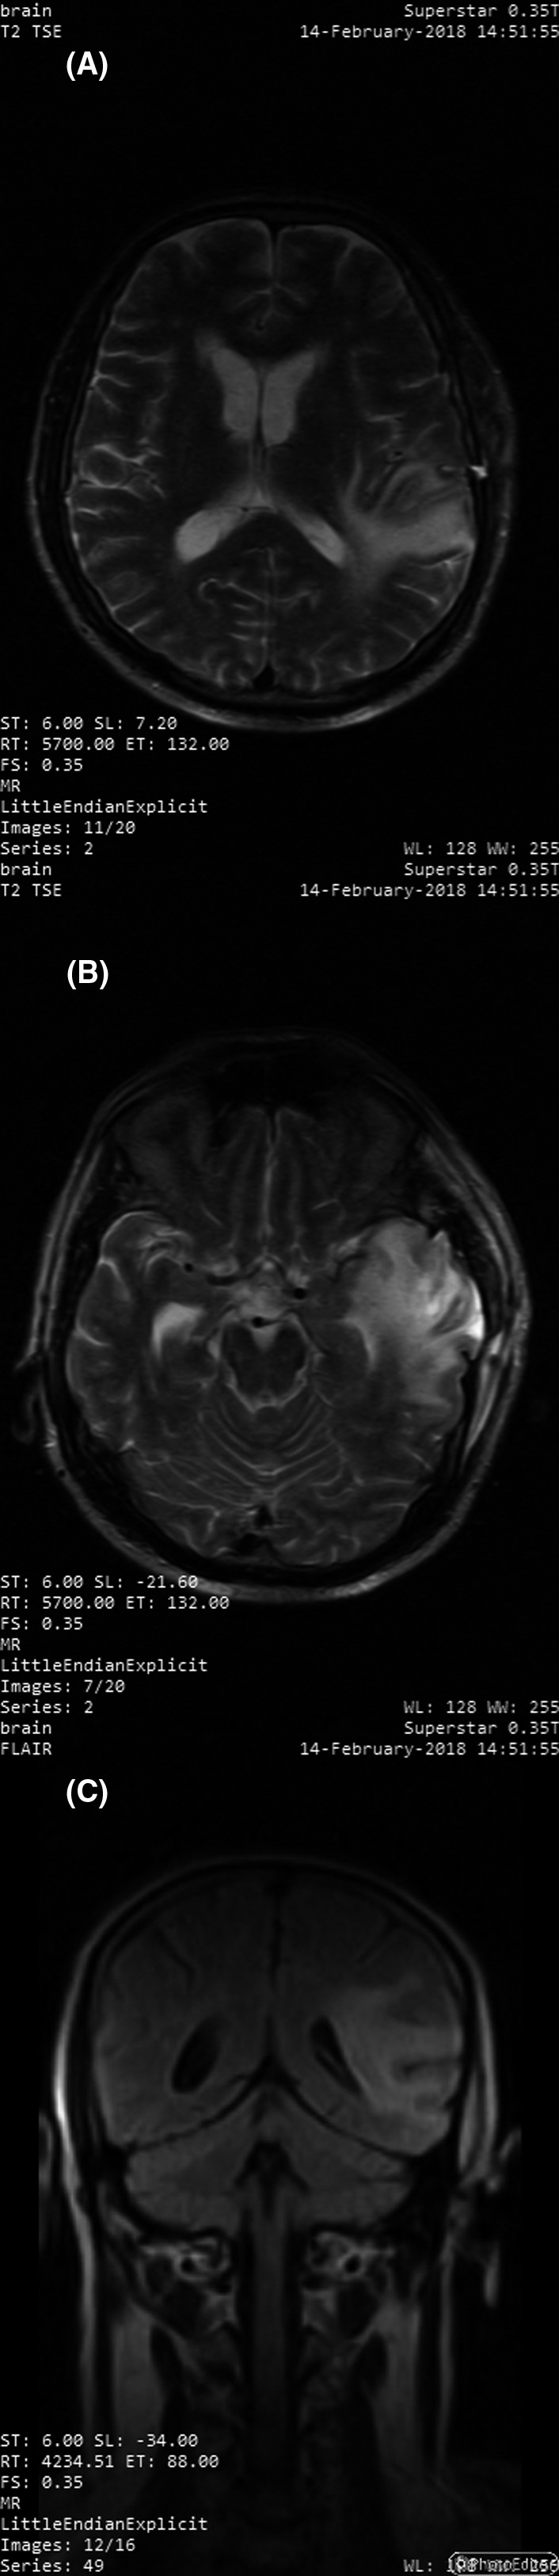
MRI 4 mo after the surgery showing the area of the lesion with residual edema with no midline deviation nor ventricular compression on transverse T2 TSE images (A and B) and coronal FLAIR image (C)
